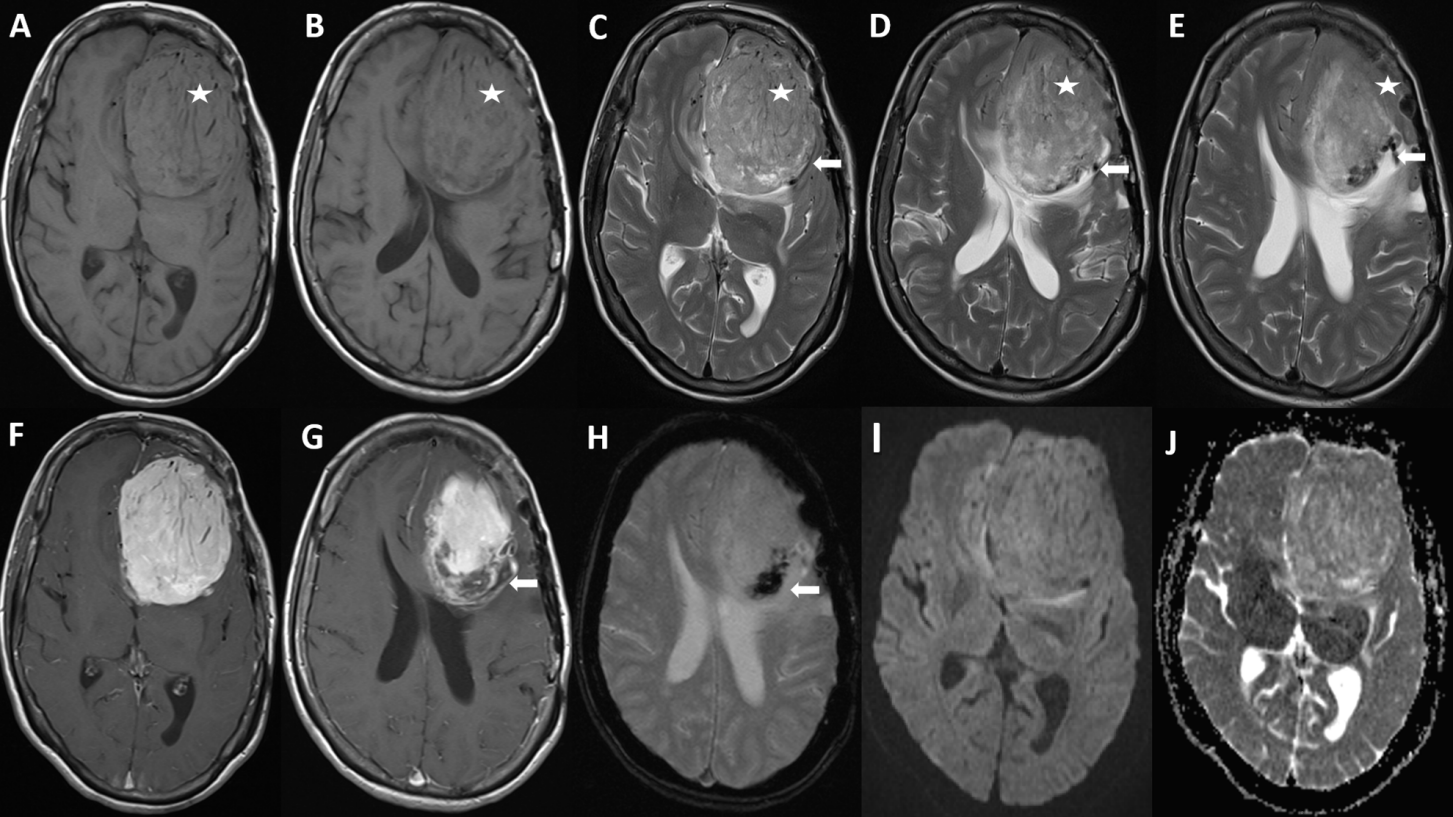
Axial *T*_1_W (A, B) and *T*_2_W (C, D, E) MR images show a large *T*_1_W isointense and heterogeneous *T*_2_W extra axial mass with low *T*_2_W signal areas (stars). The lesion shows avid enhancement following contrast administration (F, G), and a narrow zone of attachment with the dura, mushrooming centrally towards the brain parenchyma. The lesion contains multiple internal flow voids on axial *T*_2_W (C, D, E), post-contrast (G) and GRE (H) images which is consistent with a highly vascularized tumour (arrows). There is no signal change on DWI (I) and ADC (J) sequences to indicate restricted diffusion within the lesion. The constellation of MRI features is compatible with a (recurrent) intracranial haemangiopericytoma. ADC, apparent diffusion coefficient; DWI, diffusion weighted imaging; GRE, gradient echo.
